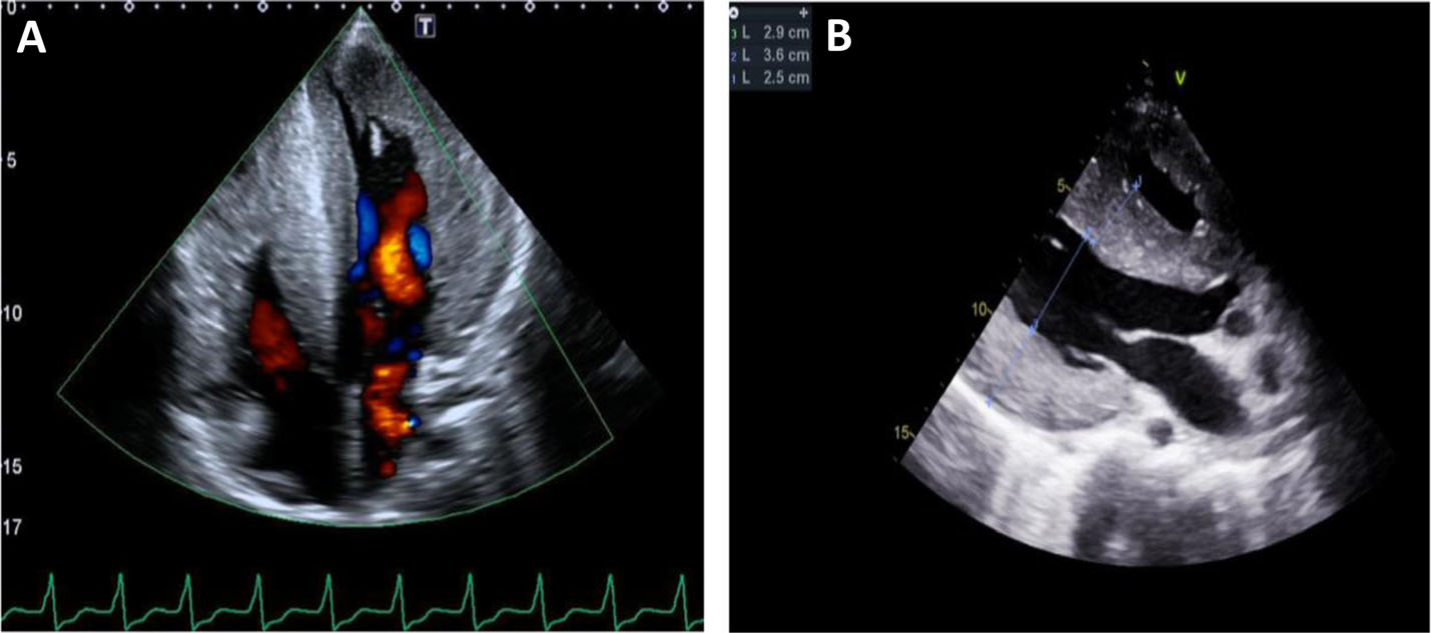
a-b Echocardiogram showing apical four chamber (A) and parasternal long-axis (B) views showing severe biventricular hypertrophy with a maximum thickness of 30 mm
